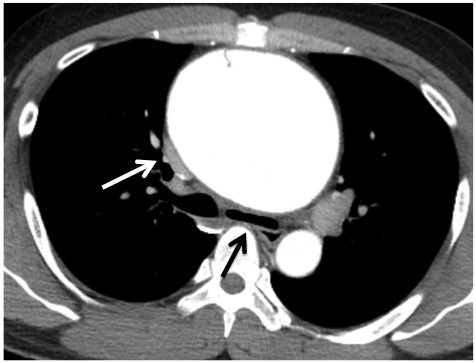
Preoperative chest CT. CT scan showing a large, thoracic aortic aneurysm (TAA) in the ascending aorta with a small aortic dissection that did not reach the sinus of Valsalva. The descending aorta was intact. There were no signs of rupture, such as pericardial effusion or hemothorax. The right pulmonary artery (white arrow) and the left main bronchus (black arrow) was compressed but not occluded.
